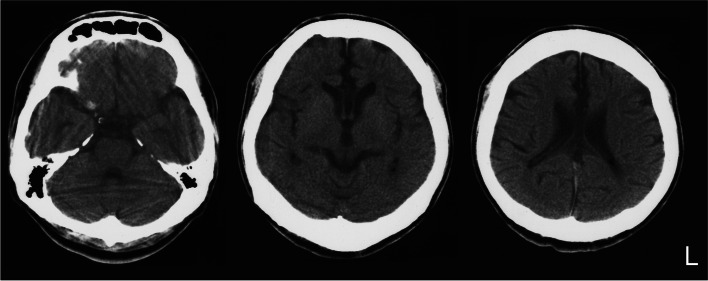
Computed tomography of the patient’s brain at the age of 24 years. The skull was thick, and the corpus callosum showed thinning. The frontal and temporal lobes were atrophic, while the cerebellum was relatively preserved
